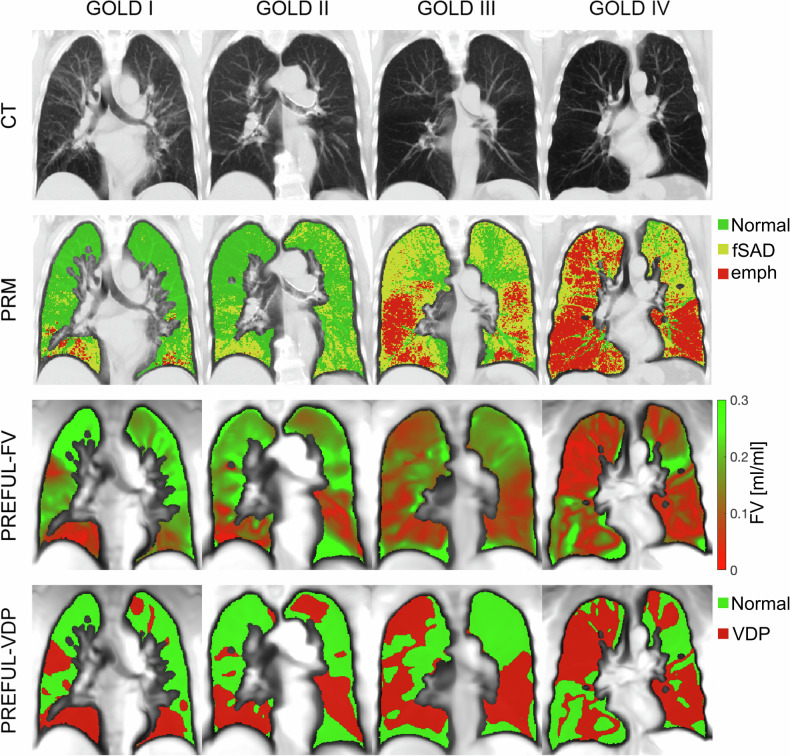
Representative corresponding coronal maps of PRM derived by CT and ventilation defect maps (PREFUL-VDP) calculated from fractional ventilation maps (PREFUL-FV) derived by PREFUL MRI. First column: a 60-year-old man with GOLD I disease (FEV_1_ 91% of predicted value, PRM^normal^ 78%, PRM^fSAD^ 19%, PRM^emph^ 4%, median PREFUL-FV 0.20, PREFUL-VDP 38%, spatial overlap 69%). Second column: a 70-year-old woman with GOLD II disease (FEV_1_ 56% of predicted value, PRM^normal^ 75%, PRM^fSAD^ 25%, PRM^emph^ 0%, median PREFUL-FV 0.20, PREFUL-VDP 39%, spatial overlap 64%). Third column: a 63-year-old woman with GOLD III disease (FEV_1_ 46% of predicted value, PRM^normal^ 25%, PRM^fSAD^ 58%, PRM^emph^ 18%, median PREFUL-FV 0.13, PREFUL-VDP 46%, spatial overlap 56%). Fourth column: a 66-year-old man with GOLD IV disease (FEV^1^ 23% of predicted value, PRM^normal^ 9%, PRM^fSAD^ 40%, PRM^emph^ 52%, median PREFUL-FV 0.07, PREFUL-VDP 58%, spatial overlap 56%)
